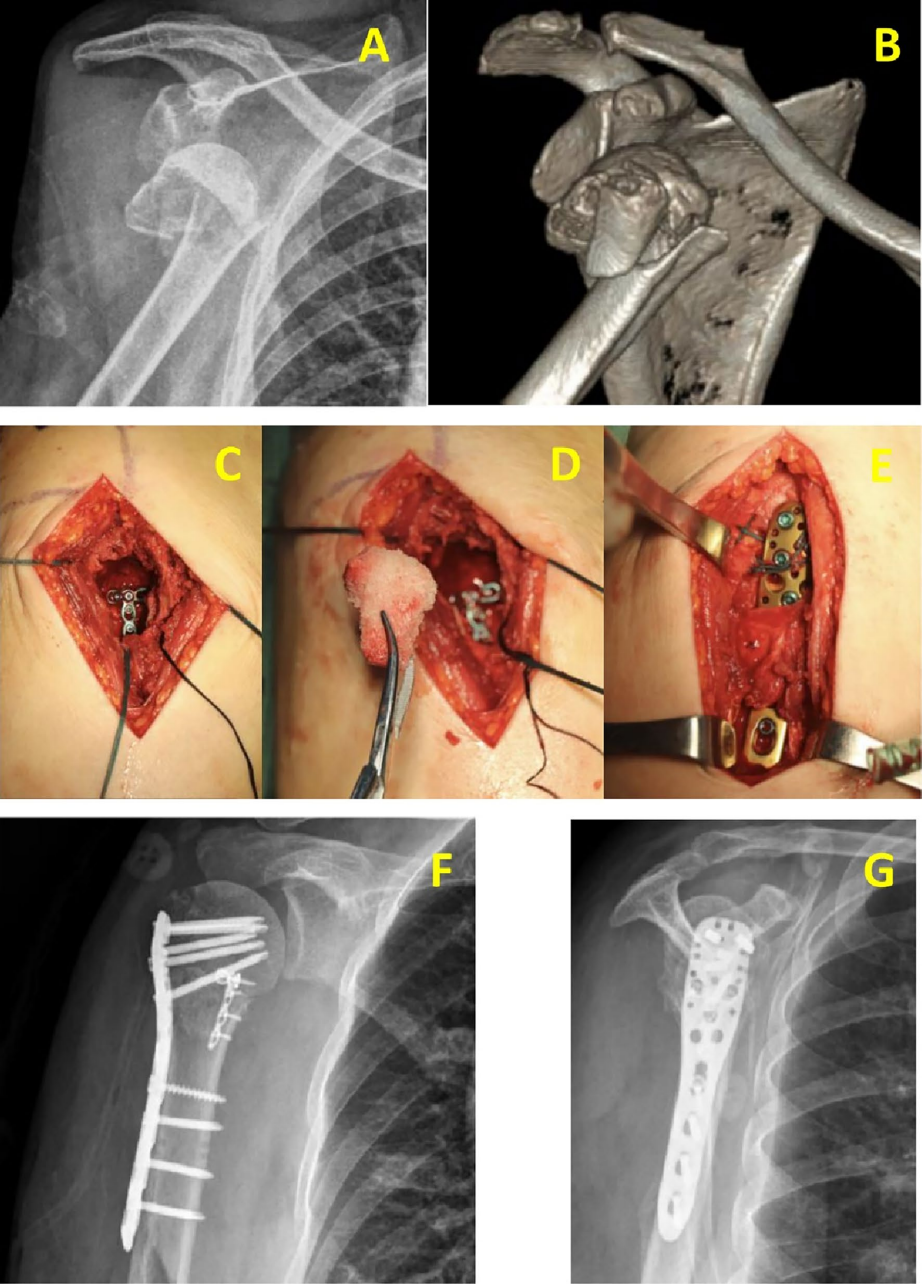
Typical case 2. An 80-year-old female patient suffered from right shoulder pain and limited mobility for 3 h due to a fall. The pre-operative X-ray and CT scan showed severe displacement of proximal humerus (A, B); The medial supporting plate was used (C); An allogenic allograft was inserted in the bony defect (D); Protection of the axillary nerve(E); Intraoperative X-ray (F, G)
